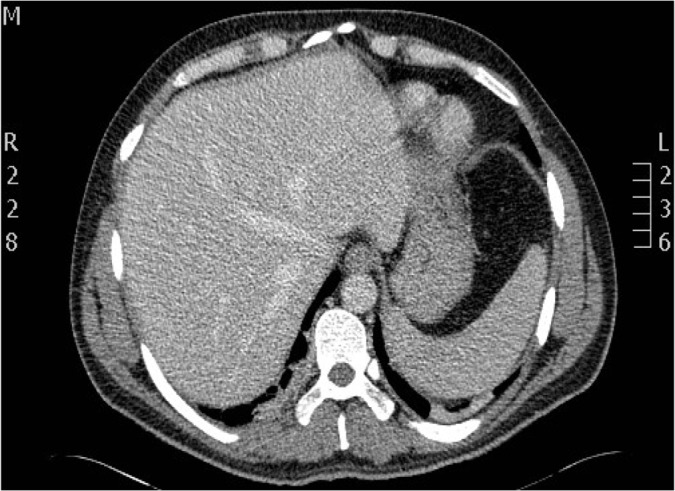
Computed Tomography of the abdomen confirming the hepato- and splenomegaly.
